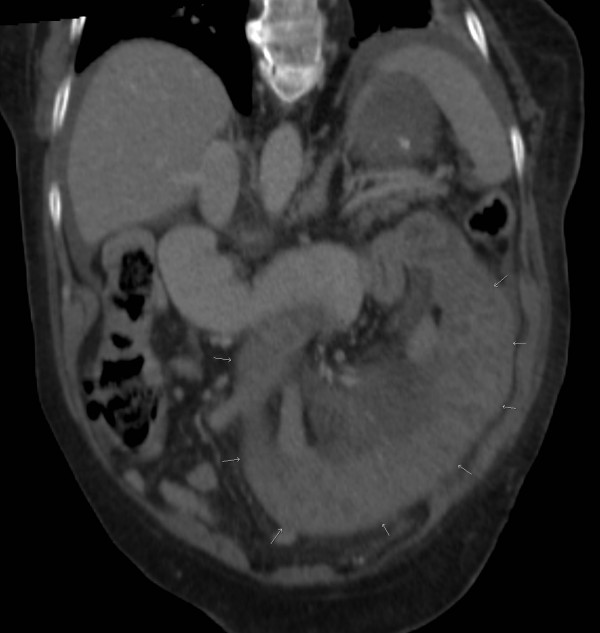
Reformatted CT scan of intramural haematoma of jejunum. This image reformatted in the coronal plane shows the full extent of the abnormal bowel (arrows). A relatively long segment of abnormal bowel (around 20 cm) is typical. There is free fluid around the spleen in the left upper quadrant.
